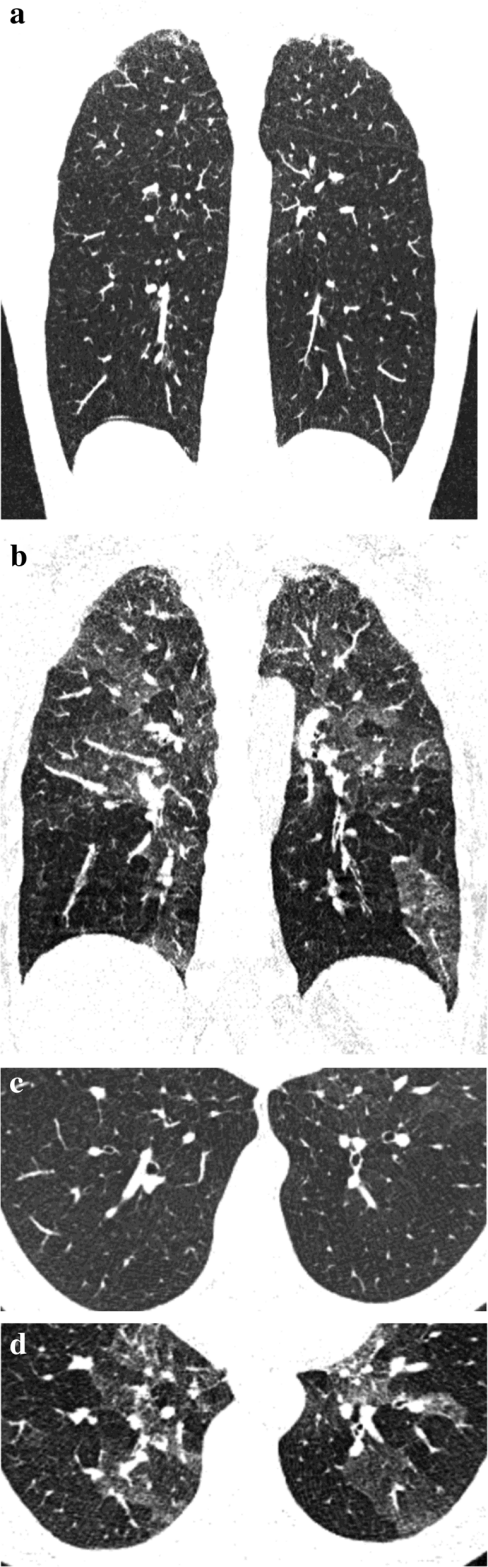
30-year-old man with chronic asthma. Coronally-reformatted inspiratory CT image shows normal lung parenchyma (a). Coronally-reformatted expiratory CT image shows extensive air-trapping (b). No collapse of trachea, main and lobar bronchi could be seen on axial scan (not shown). Axial inspiratory CT image through the lung bases shows normal bronchi (c). Axial expiratory CT scan demonstrates extreme narrowing of the bronchial lumen with air-trapping due to bronchial hyper-responsiveness (d).
